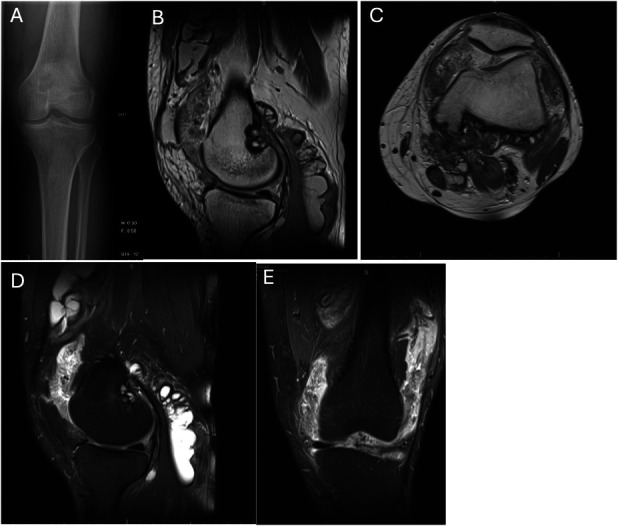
Pretreatment imaging of patient treated with both pexidartinib and surgery. Fig. 3-A Anterior posterior radiograph of the knee demonstrates mild medial joint space narrowing without osseous arthritic changes. Normal alignment. There is focal osteolysis evidence in the superior aspect of the medial femoral condyle. Fig. 3-B T1-weighted sagittal and axial (Fig. 3-C) MRI of the knee demonstrating massive intra- and extra-articular tumor burden extending from the suprapatellar pouch anteriorly to the gastrocnemius tendon bursa posteriorly. There is progression into the posterior aspect of the distal femur. Fig. 3-D T2-sagittal MRI highlighting the massive joint effusion and postcontrast enhancement (Fig. 3-E) of the diffuse synovial tumor throughout every knee compartment extending to the popliteal fossa. MRI = magnetic resonance imaging.
